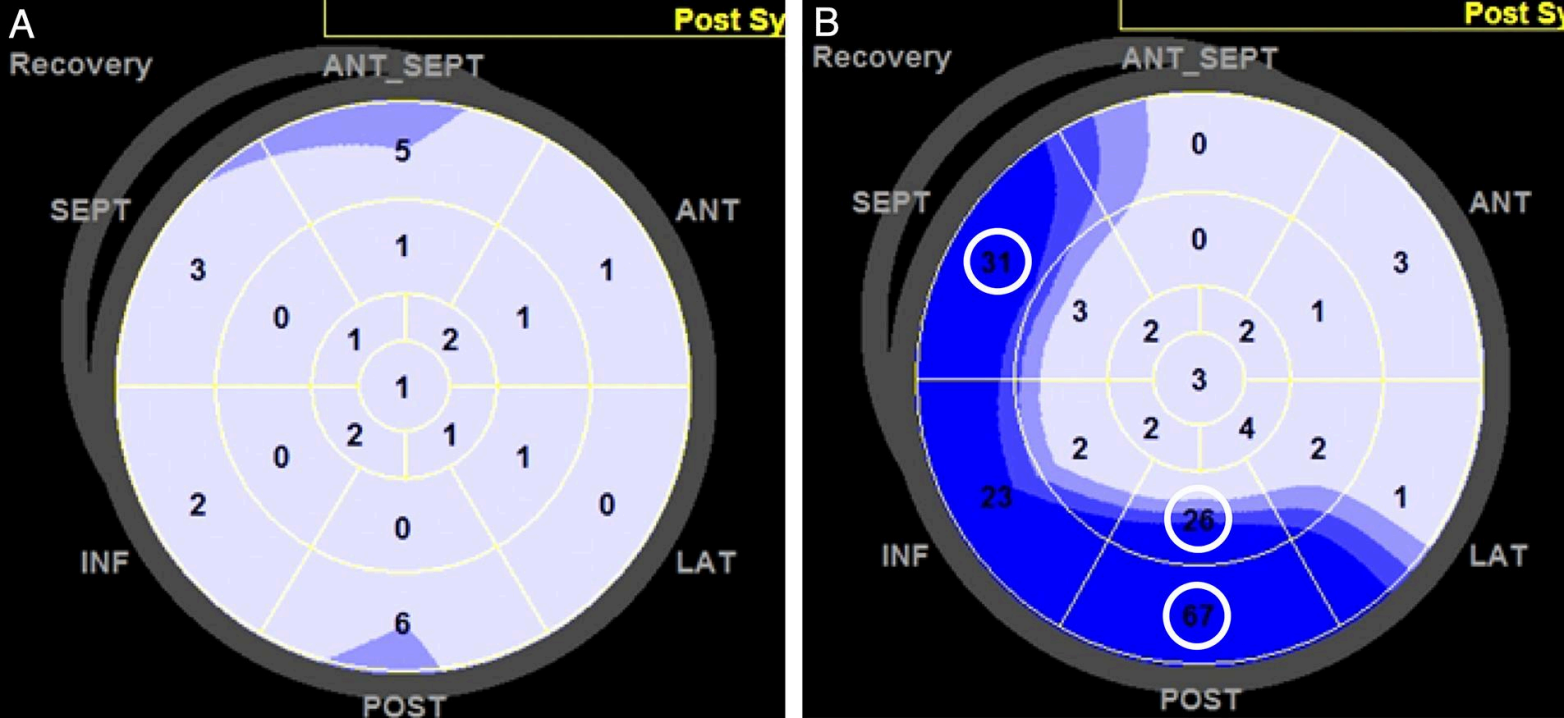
Assessment of the post-systolic index (PSI) during the recovery phase of dobutamine stress echocardiography (DSE). The PSI was automatically calculated for each left ventricular (LV) segment using software and displayed in a polar map as a percentage. PSI values were considered positive if two or more adjacent LV segments had PSI values exceeding the predefined cutoff of 25%. (A) Example of a patient with a negative PSI during the recovery phase, where all LV segments had low PSI values. (B) Example of a patient with a positive PSI, where several LV segments (circled) exhibited PSI values ≥25.
